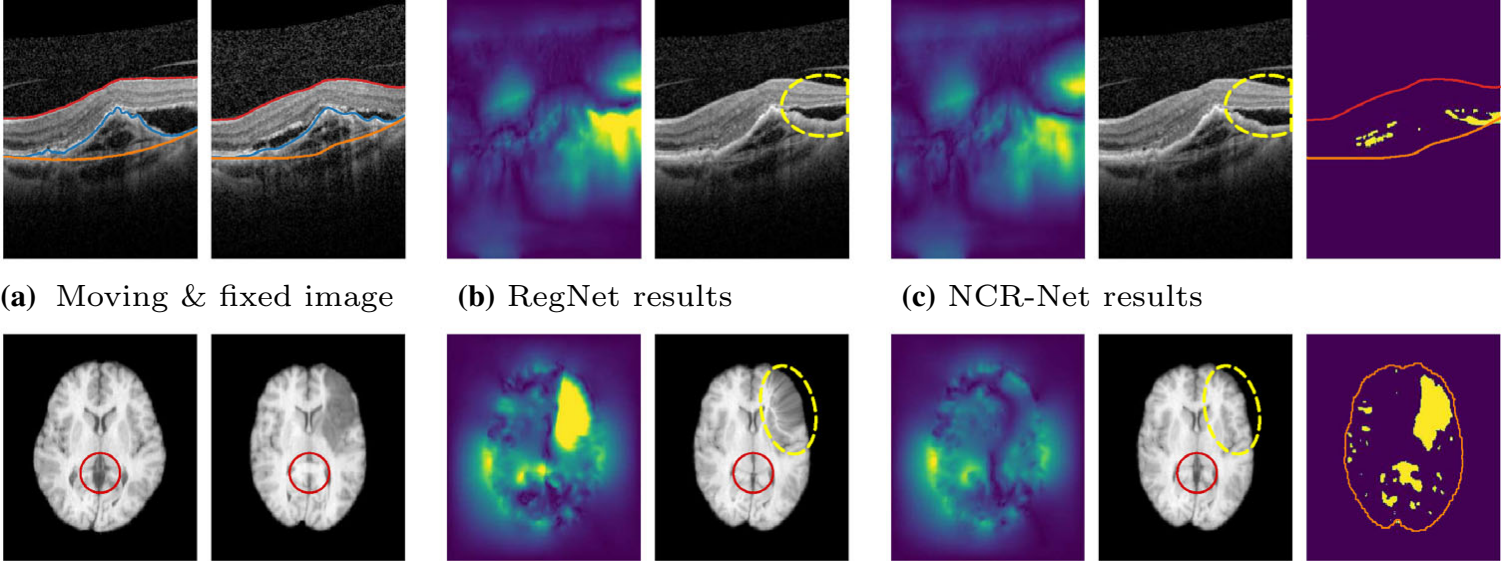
Results of RegNet and NCR-Net$$_\mathrm {s}$$ for the registration of OCT B-scans (upper row) and MRI slices (lower row). ILM, RPE and BM are marked in the OCT images with red, blue and orange lines, respectively. In (b) and (c) deformation fields, deformed moving images and, if applicable, segmentations of non-correspondent image regions are shown. The non-correspondence segmentation of NCR-Net allows for more plausible deformations in pathological (yellow dashed ovals) and non-correspondent (red circles) areas
